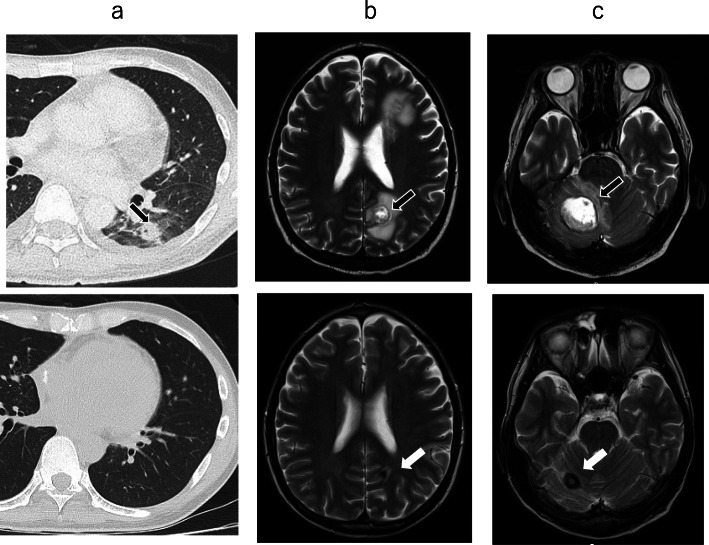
Pre- and postoperative chest computed tomography findings and brain magnetic resonance imaging findings. Preoperative contrast-enhanced chest computed tomography revealed nodular lung shadows with a small cavity in the left lower lobe (a—upper: arrow). Chest computed tomography performed at 3 months after surgery revealed no nodular lung shadows in the left lower lobe (a—lower). Magnetic resonance imaging demonstrated multiple bilateral rim-enhancing lesions with surrounding vasogenic edema in the left parietal lobe (b—upper: black arrow) and the cerebellum (c—upper: black arrow). The brain abscesses in the left parietal lobe (b—lower: white arrow) and cerebellum (c—lower: white arrow) had healed on the magnetic resonance imaging performed at 1 year after surgery
